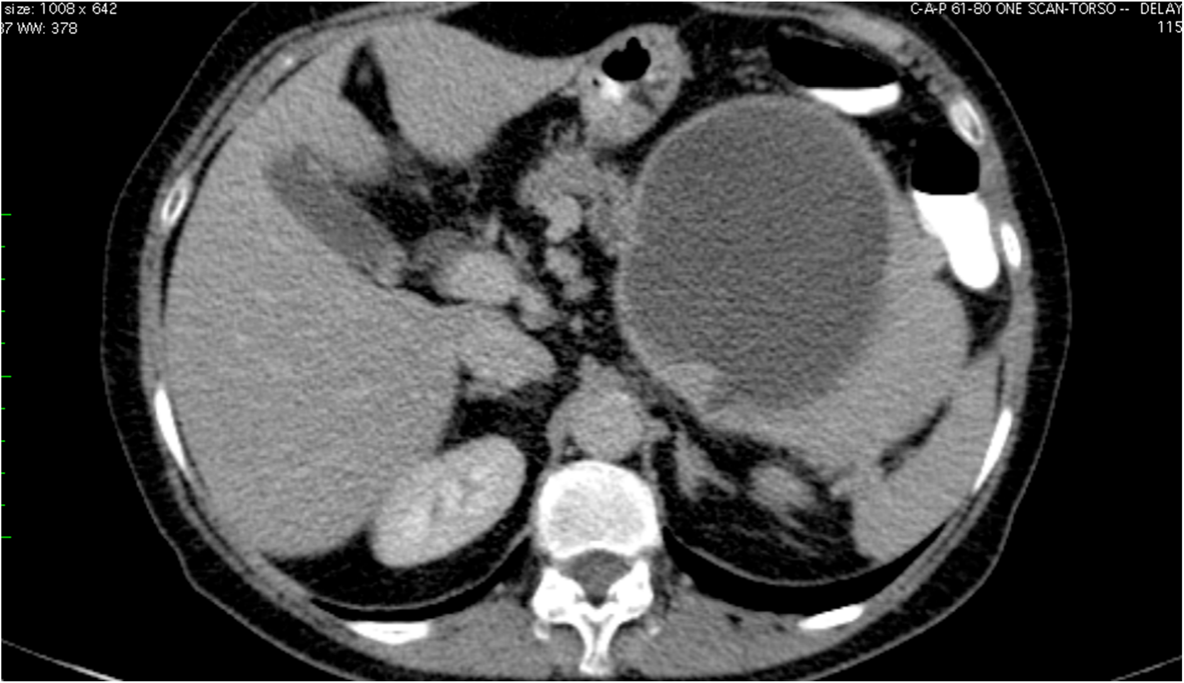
Enhanced computed tomography (CT) shows the presence of a cystic lesion in the tail of the pancreas.
